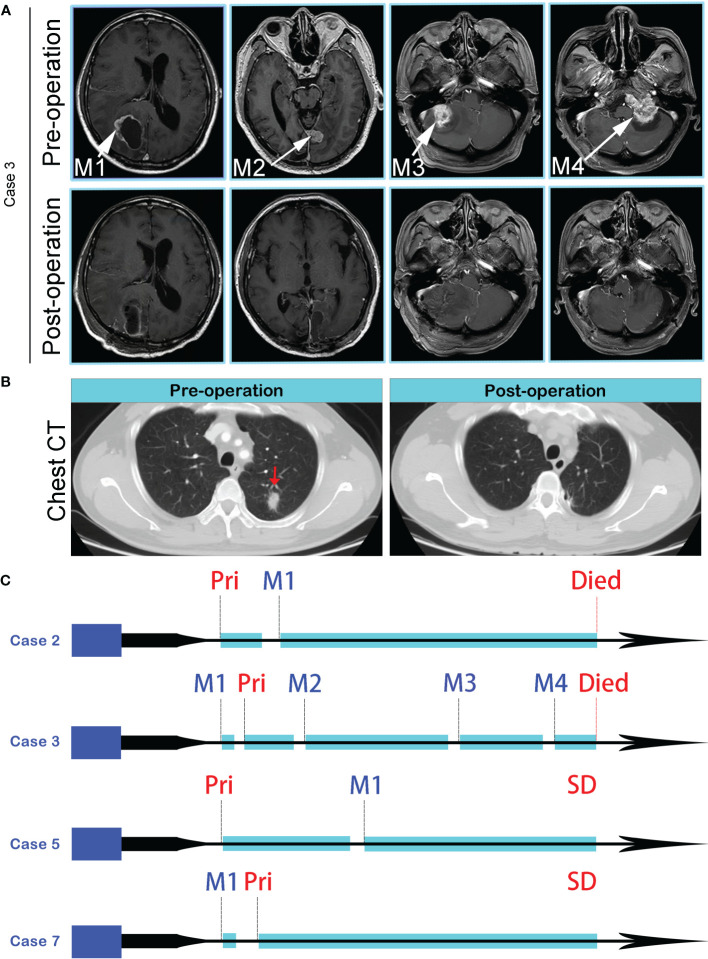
(A) MR images of four spatial and temporal isolated BMs in Case 3, before (the upper panel) and after (the lower panel) surgeries. (B) CT imaging revealed an isolated lung lesion (Red arrow), and postoperative chest CT showed the lesion was removed totally. (C) Disease progression and treatment timelines of Case 2, Case 3, Case 5, and Case 7. Pri, Primary lung tumor; SD, Stable Disease.
